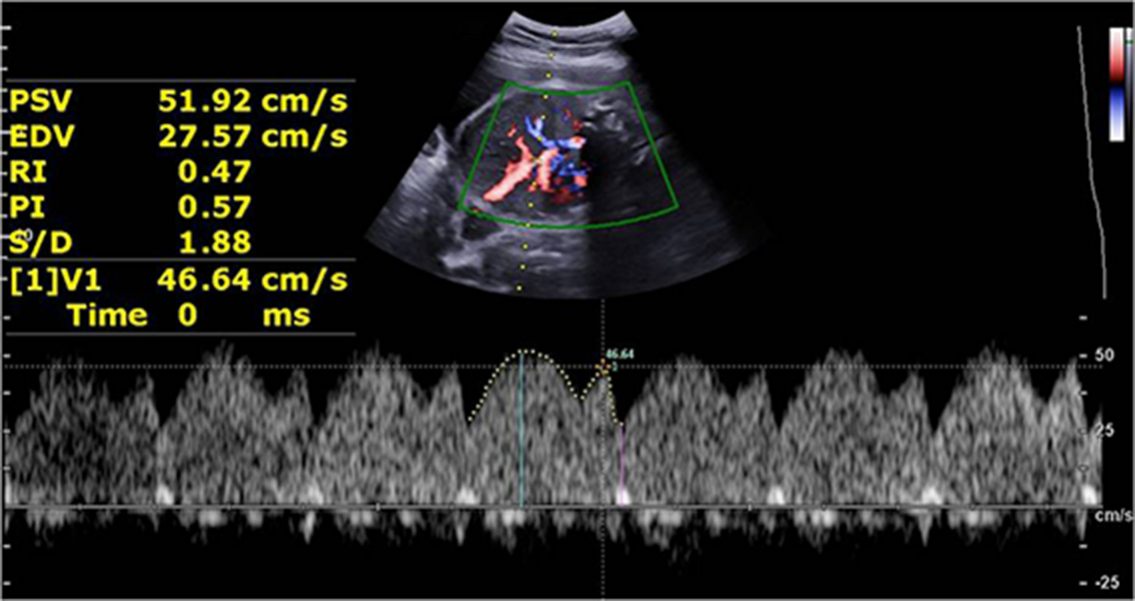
Doppler measurement of the DV. Abbreviations are as in Figure 4.
